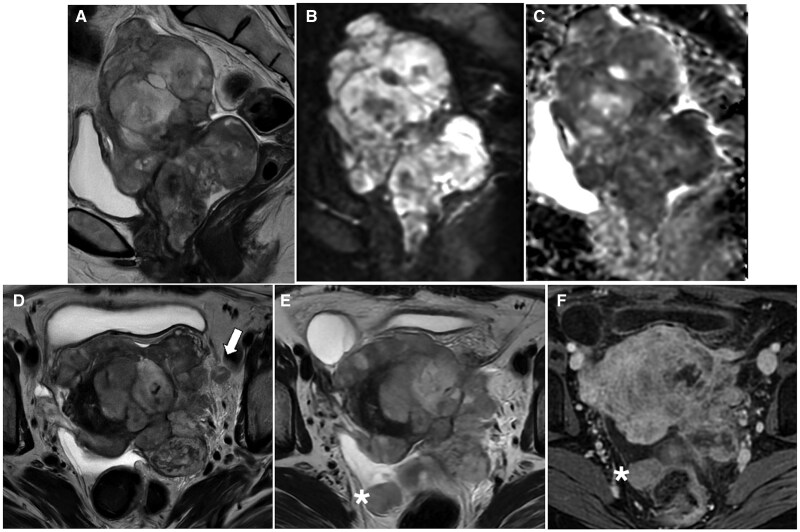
Cervical carcinosarcoma. MRI shows a large lesion replacing the normal uterine anatomy, with heterogeneous appearance on T2-WI (A, D, E) and marked impeded diffusion (B, C). There is also evidence of nodal (arrow in D) and peritoneal metastases (asterisks in E and F), reflecting the aggressive nature of carcinosarcoma.
